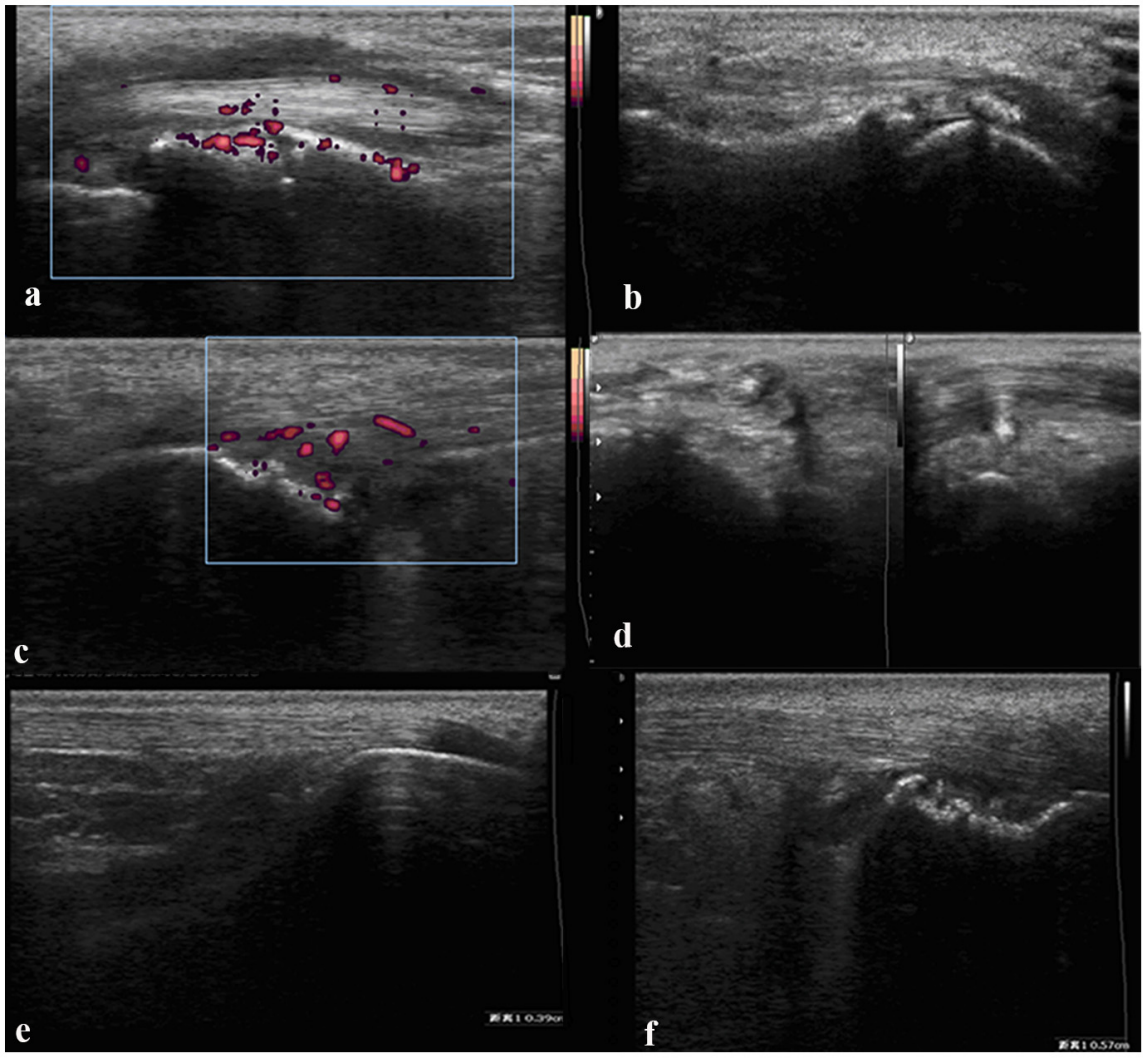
US abnormalitis in entheses. a shows tendon sheath with fluid, vacularization and synovial proliferation. b shows marked calcific insertional tendinopathy. c shows the signal of power doppler which is located inside the calcaneal tendon and tendon thickening. d demonstrates anterior tibial tendon with hypoechogenicity. e and f show the Achilles tendon of the same patient. e demostrates the normal Achilles tendon. f shows tendon thickening and calcaneal erosions.
